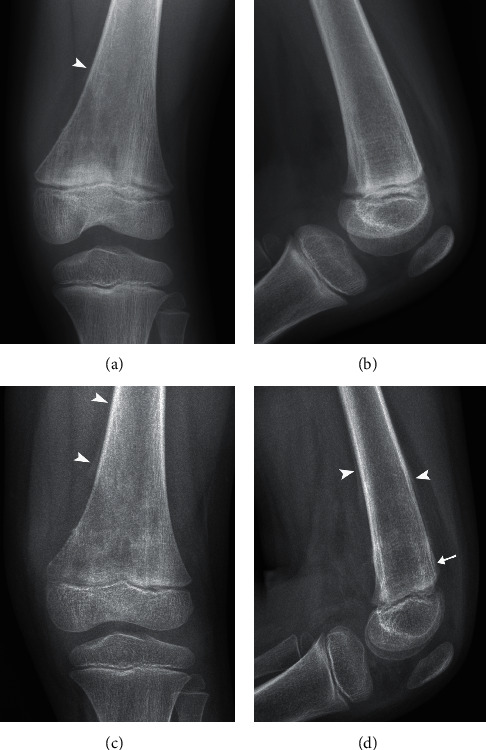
Anteroposterior and lateral knee radiographs at presentation (a, b) and 15 days later (c, d) show interval progressive medullary-based eccentric lytic change with a wide zone of transition, aggressive (Codman's triangle) periosteal reaction (arrowheads), and subtle cortical deformity (arrow).
